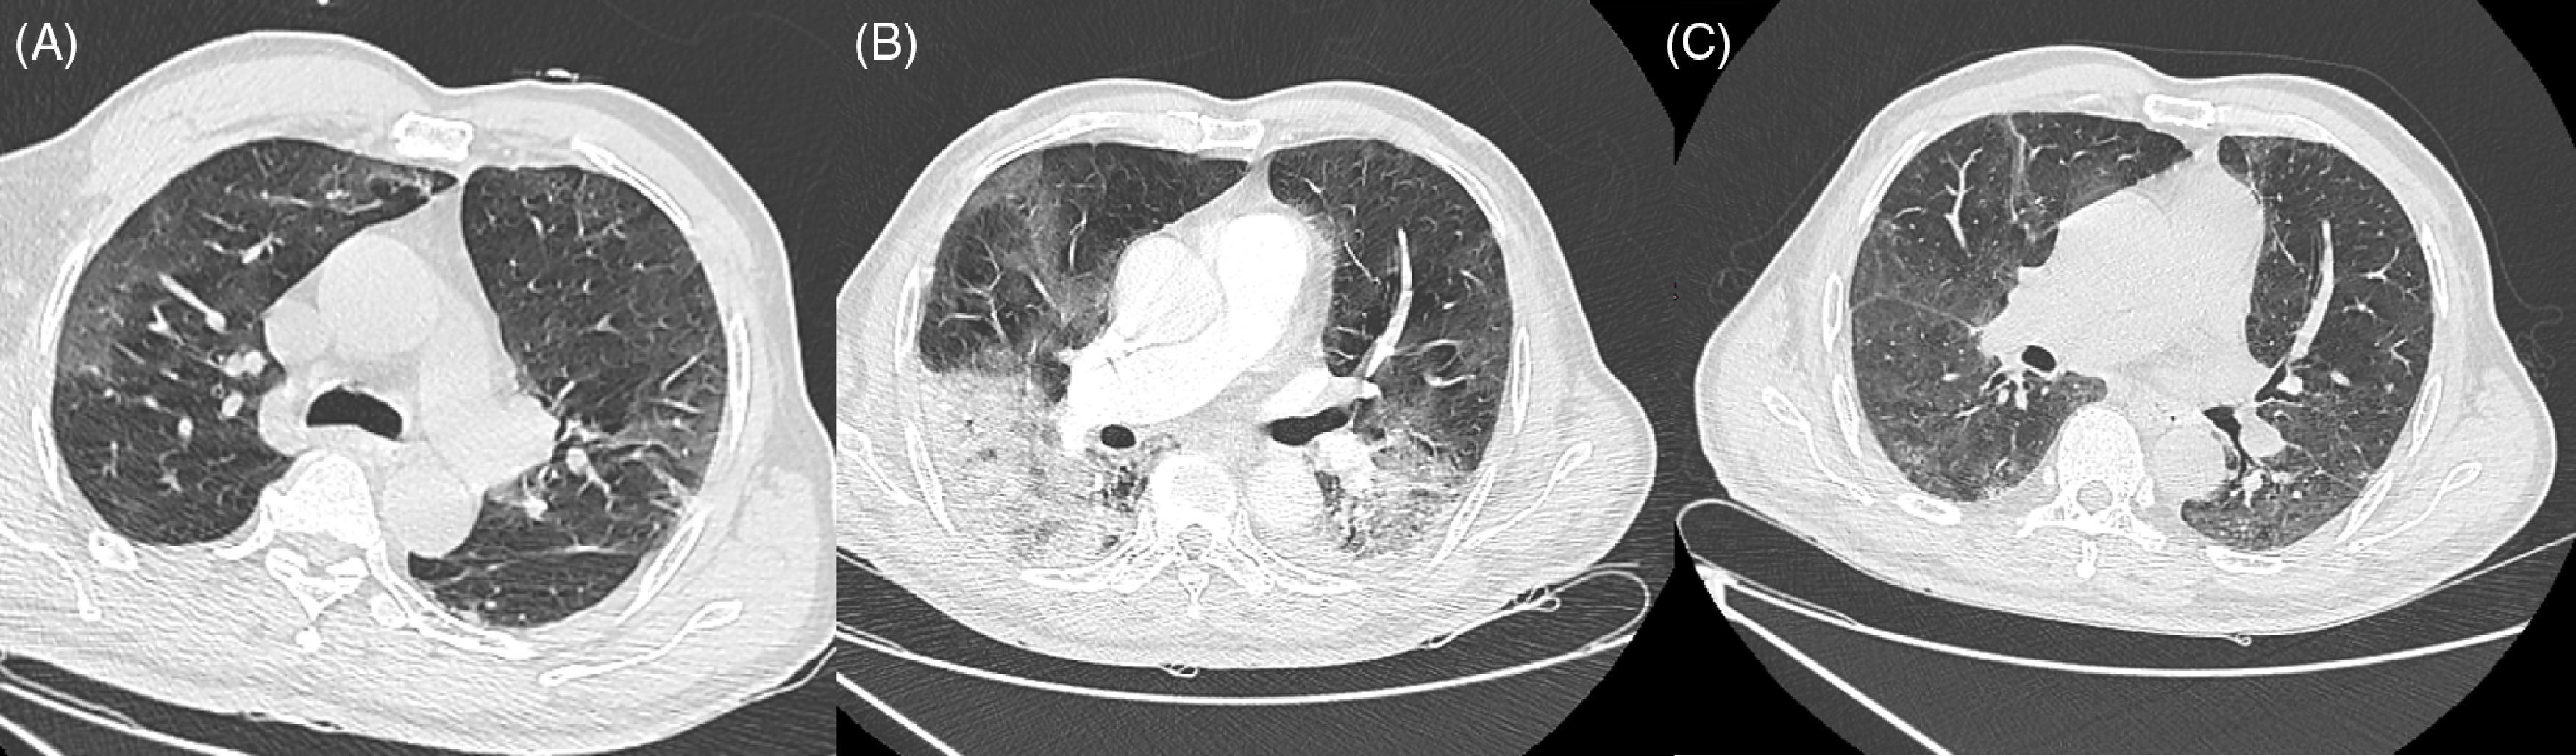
Lung CT Imaging Showing Pulmonary Lesions on Day 1 (A), Day 10 (B), and Day 52 (C).
